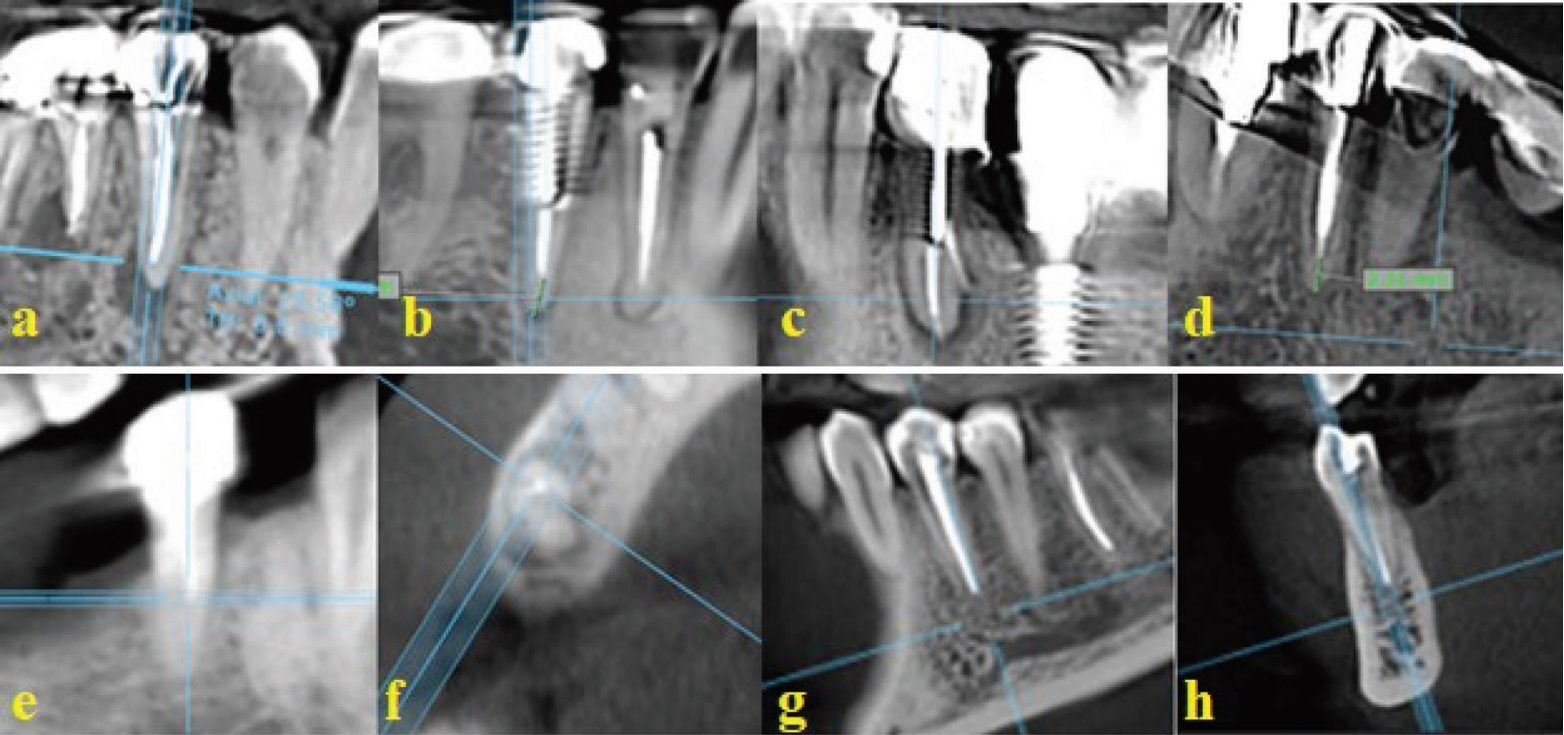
CBCT images demonstrating various endodontic procedural errors and anatomical variations: (a) coronal view of tooth #35 without any procedural error; (b) coronal view of tooth #35 with underfilling; (c) coronal view of tooth #35 with a perforation; (d) coronal view of tooth #35 with a broken instrument; (e) coronal view of tooth #45 with a missed lingual canal; (f) axial view of tooth #45 with a missed lingual canal; (g) coronal view of tooth #34 with overfilling; and (h) sagittal view of tooth #34 with overfilling.
